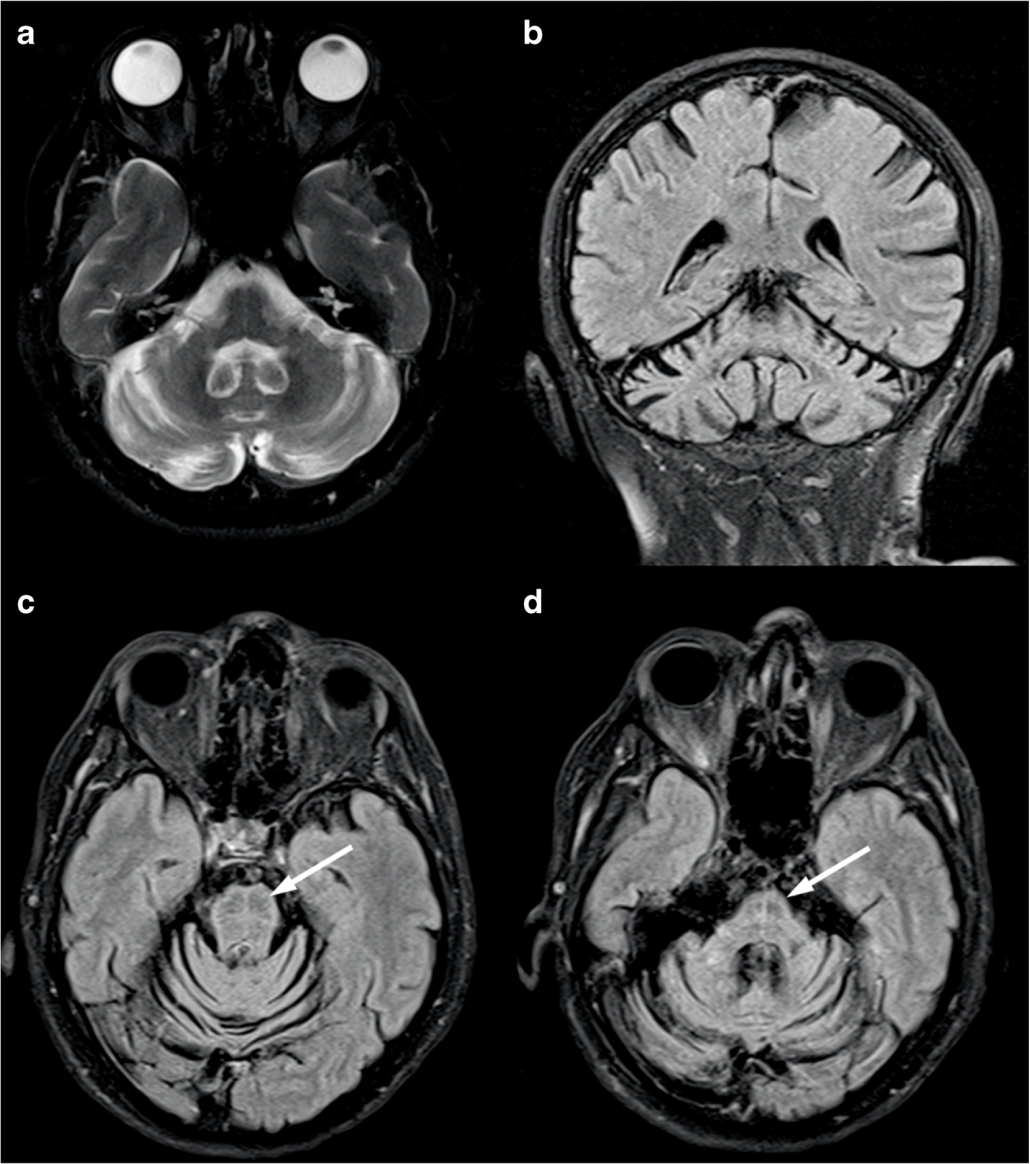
Brain MRI scan of a 69-year-old female SCA1 patient. Axial T2-weighted (a) and coronal FLAIR (b) images show a global cerebellar volume loss, along with brainstem atrophy (c–d) and the presence of the “hot cross bun” sign (arrows)
